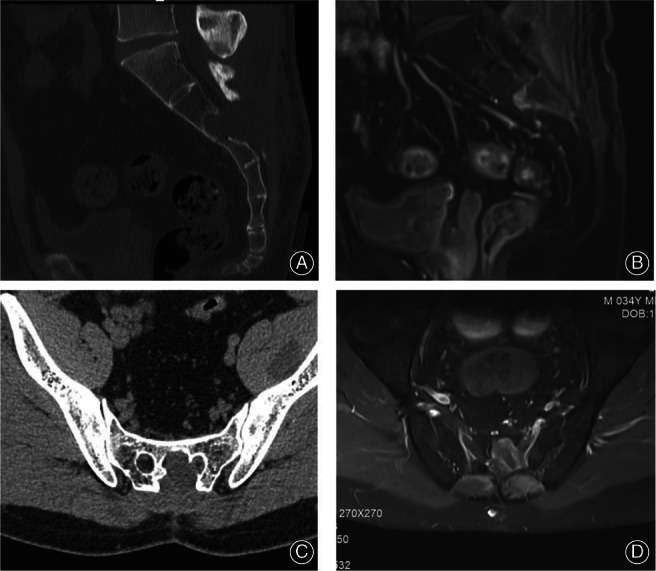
Both the sagittal postoperative CT scan and MRI showed extended surgical margin was achieved. (A and B); Both the transverse CT scan and MRI showed no sign of recurrence at the most recent follow up (C and D)
